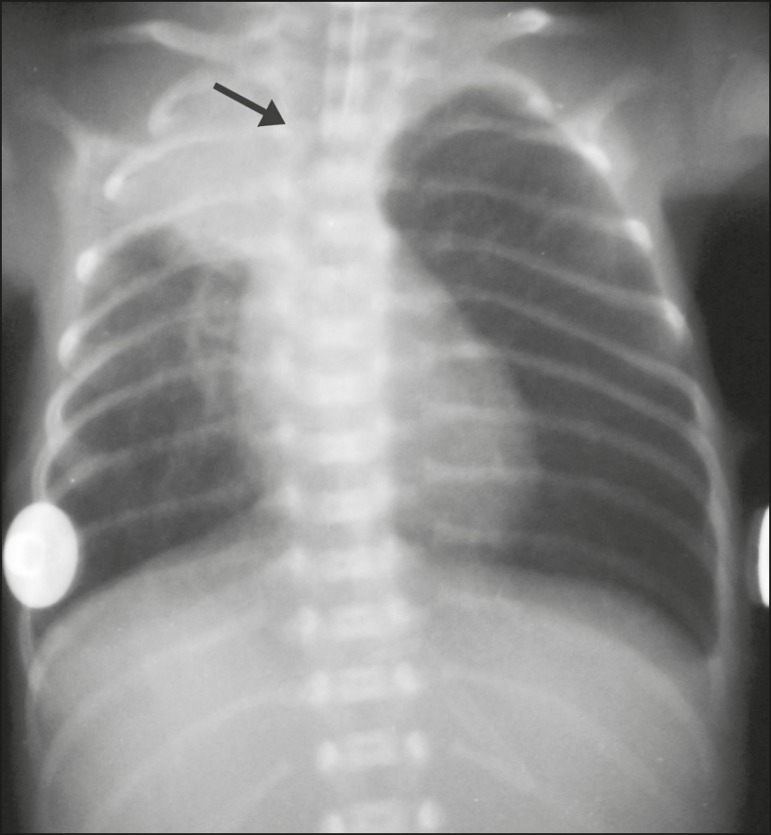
Chest X-ray of a neonate born at 25 weeks of gestation with a birth
